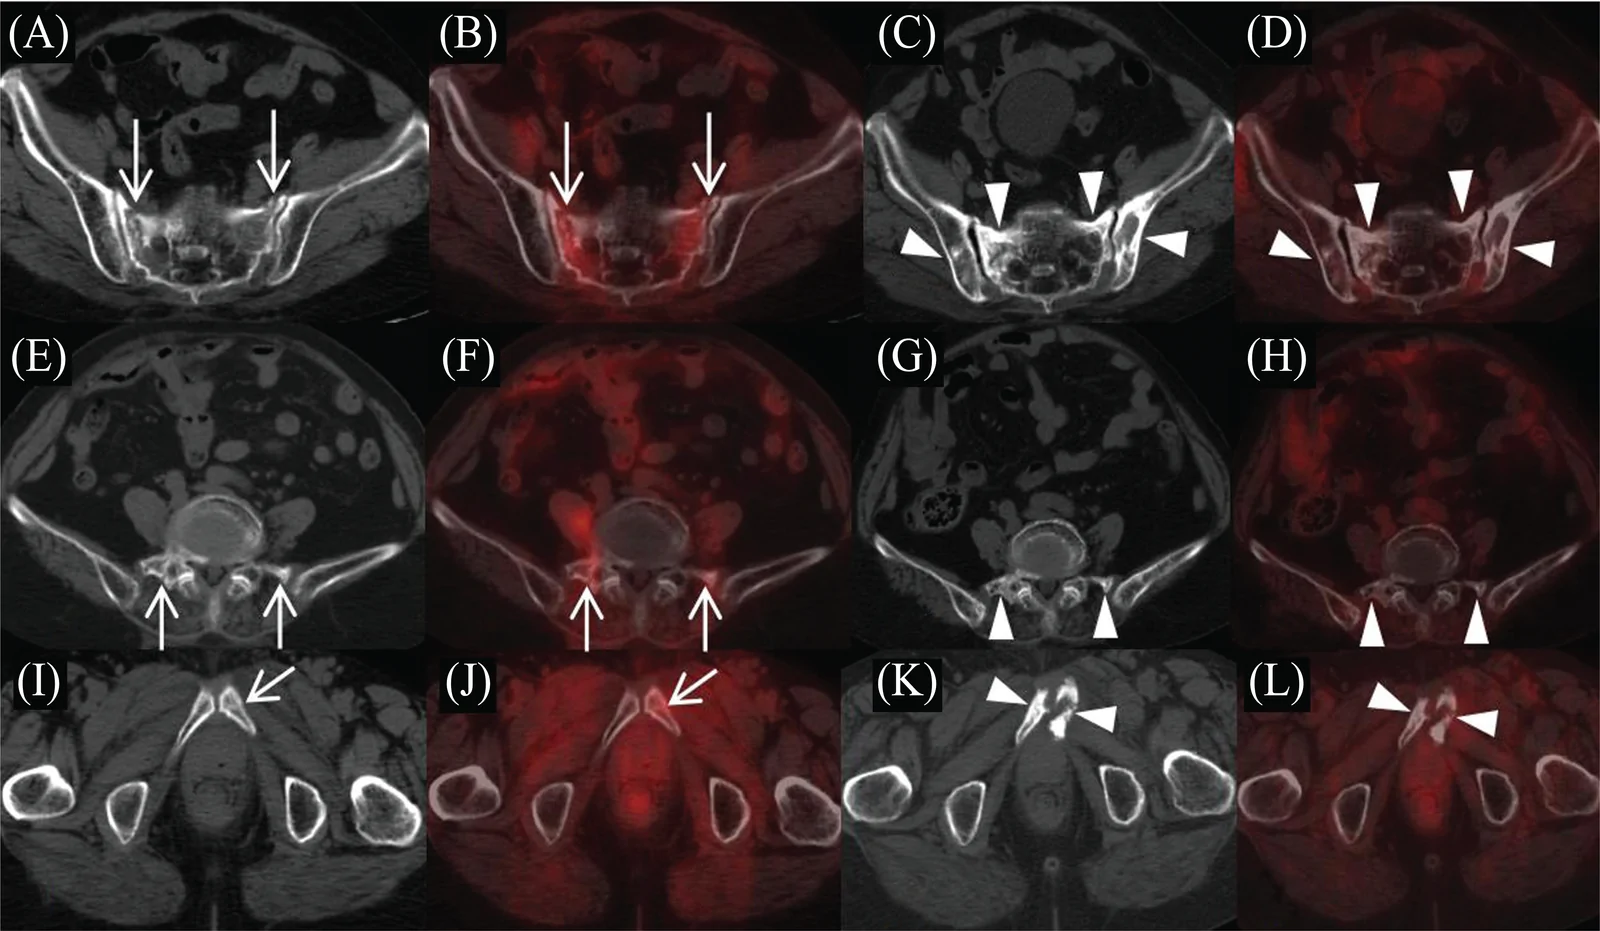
A 72-year-old female with SIFs at 9 months after radiotherapy for cervical cancer, presenting with low back pain for more than 2 months. Axial CT images (A, E, I) showed low-density fracture lines or cortical buckling in the bilateral sacral alae, bilateral transverse processes of L5, left pubic ramus, and pubic symphysis; among these, the fracture lines in the sacral alae were serrated and parallel to the sacroiliac joints (arrow ↑). Multiple ill-defined, patchy, slightly hyperdense shadows were observed around the fracture lines. The corresponding axial PET/CT fusion images (B, F, J) demonstrated diffusely mild increased FDG uptake with a SUVmax of 3.4 (arrow ↑). At the follow-up examination at 32 months after radiotherapy, axial CT images (C, G, K) revealed that the range of abnormal bone changes had significantly enlarged compared with the previous findings, showing multiple punctate and patchy hyperdense shadows with obvious proliferative sclerosis and clear boundaries in the bilateral regions.Sacral alae, bilateral ilia, bilateral transverse processes of L5, bilateral pubic rami, and pubic symphysis; only the fracture ends of the left pubic ramus and pubic symphysis were obviously separated, while the fracture lines in other locations had disappeared, showing post-repair changes (arrow ▲). The corresponding axial PET/CT fusion images (D, H, L) showed a decreased SUVmax of 1.4 (arrow ▲).
